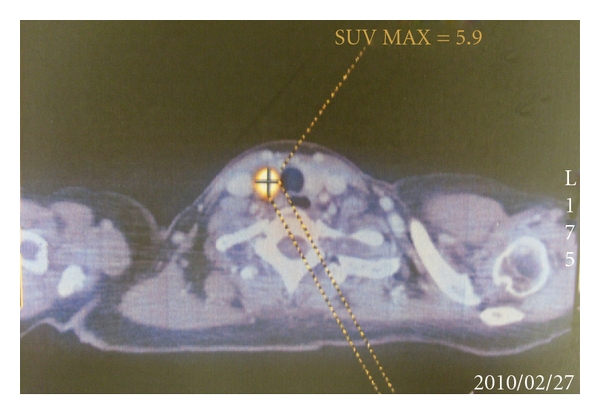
A 70-year-old lady underwent 18F-FDG PET for staging of a rectal carcinoma. A focal right thyroid hypermetabolic uptake (with a SUVmax of 5.9) was detected. This was later confirmed as papillary thyroid carcinoma (PTC) on cytology. A total thyroidectomy was performed revealing a 1.3 cm tall cell variant of PTC with central compartment lymph nodes metastases (pT1N1a).
